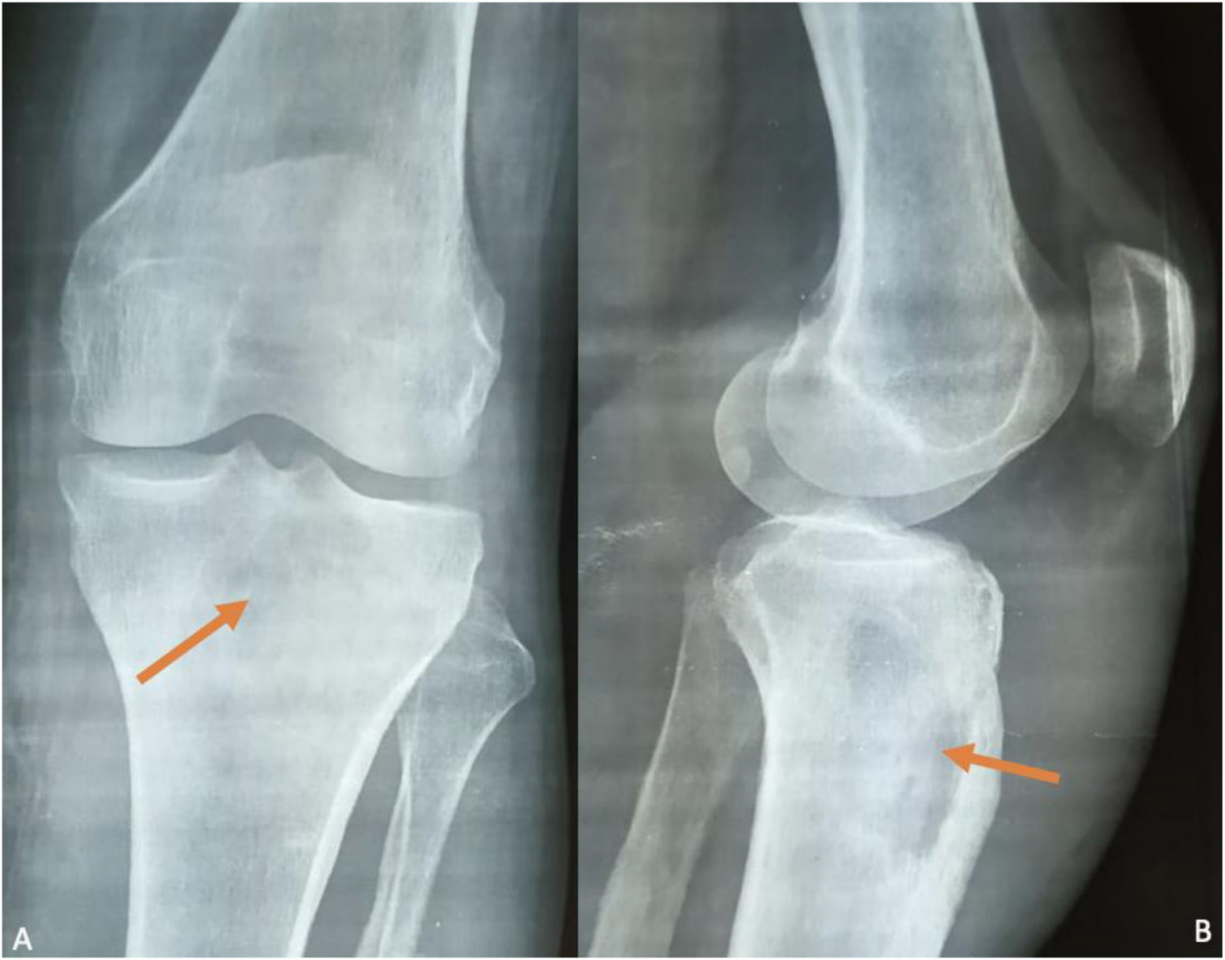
Plain radiographs of the knee, anteroposterior (A) and lateral views (B), show a well-defined pathological metaphyseal tibial lytic lesion (orange arrow) associated with marked peripheral sclerotic reaction.
